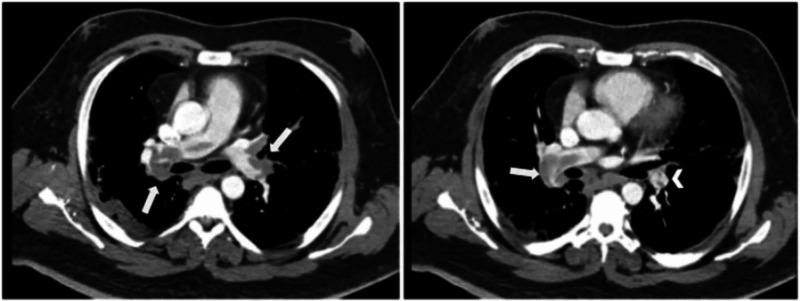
Computed tomography angiography (CTA) of the chest Axial view of the thorax showing pulmonary embolism affecting the bilateral main pulmonary arteries (white arrows). The partial filling defect surrounded by contrast material produces a polo mint sign, in keeping with acute embolus (arrowhead).
